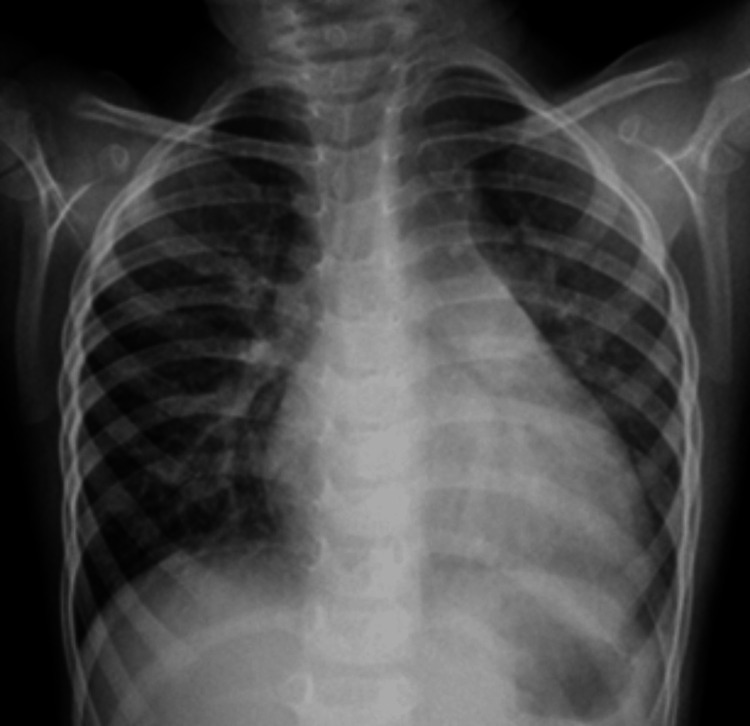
Plain chest radiography frontal view showing cardiomegaly with widening of the superior mediastinum
